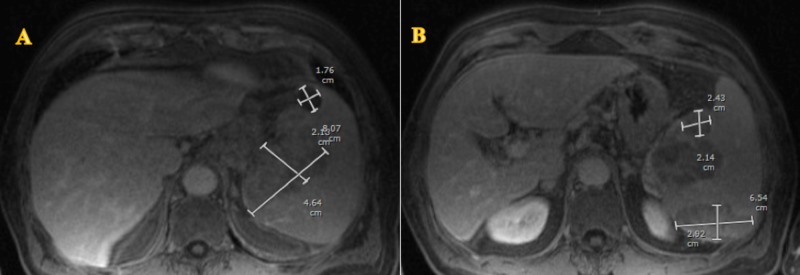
(A & B) Magnetic resonance imaging (MRI) of the abdomen showing splenomegaly with multiple hypoenhanced lobulated masses.
